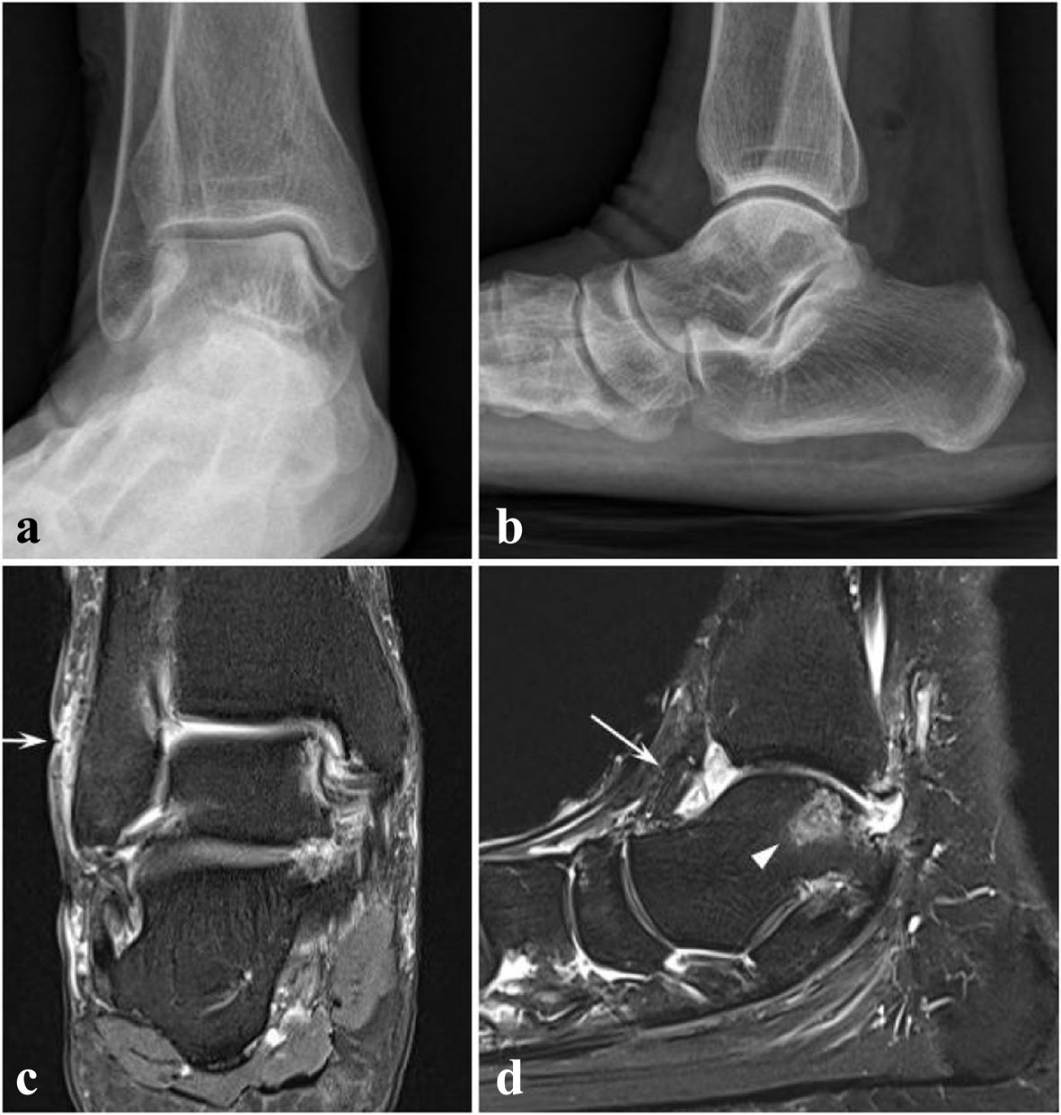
Plain radiographs and MRI of initial outpatient clinic visit. Plain radiographs (a, b) showing no significant changes from the initial images of outside hospital. Coronal view of proton density fat suppression image (c) showing thickened skin with focal defect over lateral malleolus (white arrow) with high signal intensity of subcutaneous layer, suggesting lateral malleolar bursitis with fistula. Sagittal view of T1-weighted double fat suppression images (d) showing focal bone marrow edema in talus (white arrow head) and synovial hypertrophy with effusion (white arrow) in right ankle joint, which was interpreted as nonspecific synovitis, with differential diagnosis of inflammatory arthritis, infective arthritis such as pyogenic or early tuberculous arthritis and reactive arthritis associated with ankle instability
